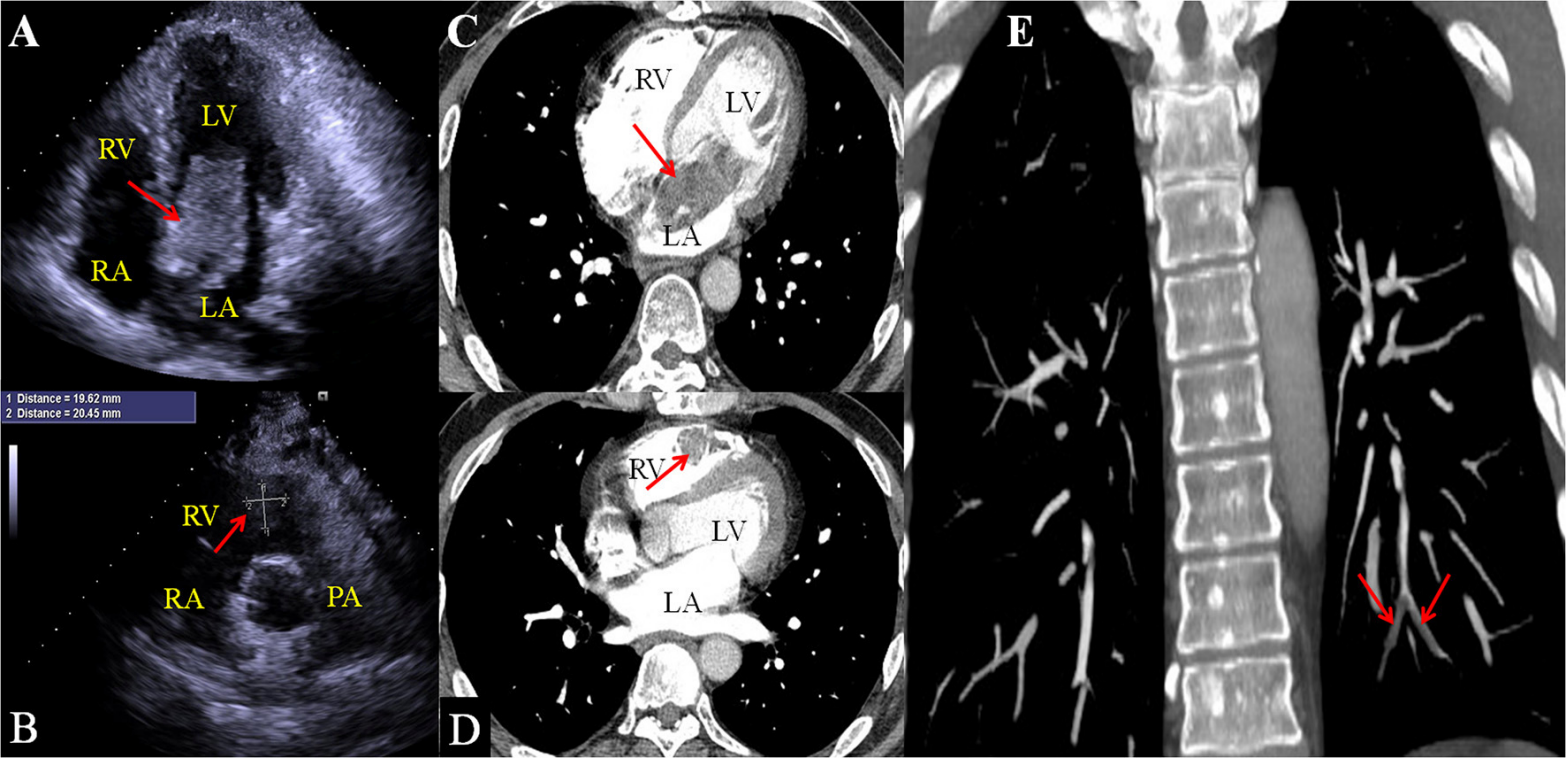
2D echocardiography shows an irregular hyper-echoic mass in the left atrium (A) and a hypoechoic mass in the right ventricular outflow tract (B). Enhanced chest CT confirms the LA mass (C) and right ventricular mass (D). CT Pulmonary arteriography confirms that the nodules on regular CT are pulmonary emboli [(E), red arrows].
